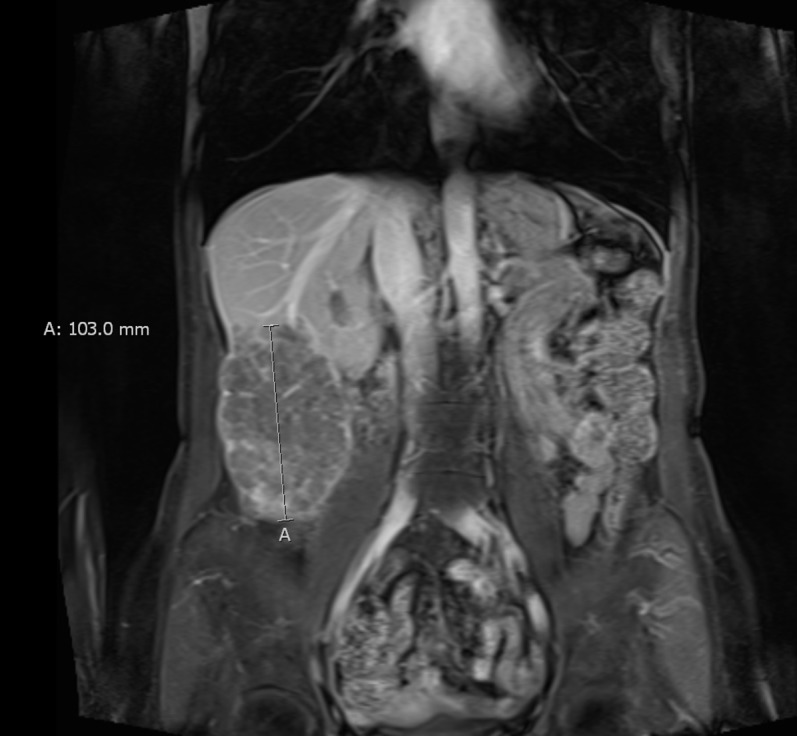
Representative coronal slice from pre-treatment MRI
